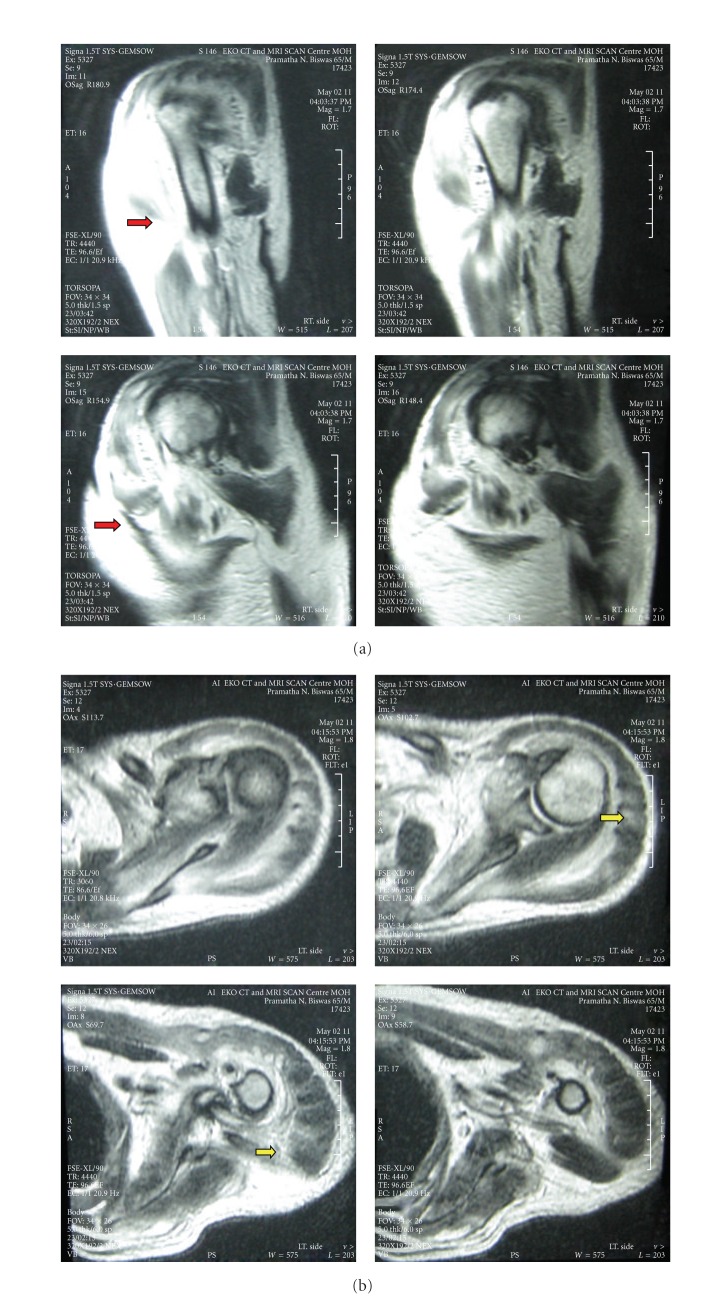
(a) Magnetic Resonance Imaging (T2W) of muscles around the right shoulder joint and right anterior chest region showing areas of increased signal intensities with areas of myositis, muscle swelling (red arrows), and subcutaneous edema. (b) Magnetic Resonance Imaging (T2W) of the muscles around the left shoulder joint revealing areas of hyperintensities suggestive of necrosis (yellow arrows) and inflammation of muscles with subcutaneous edema.
